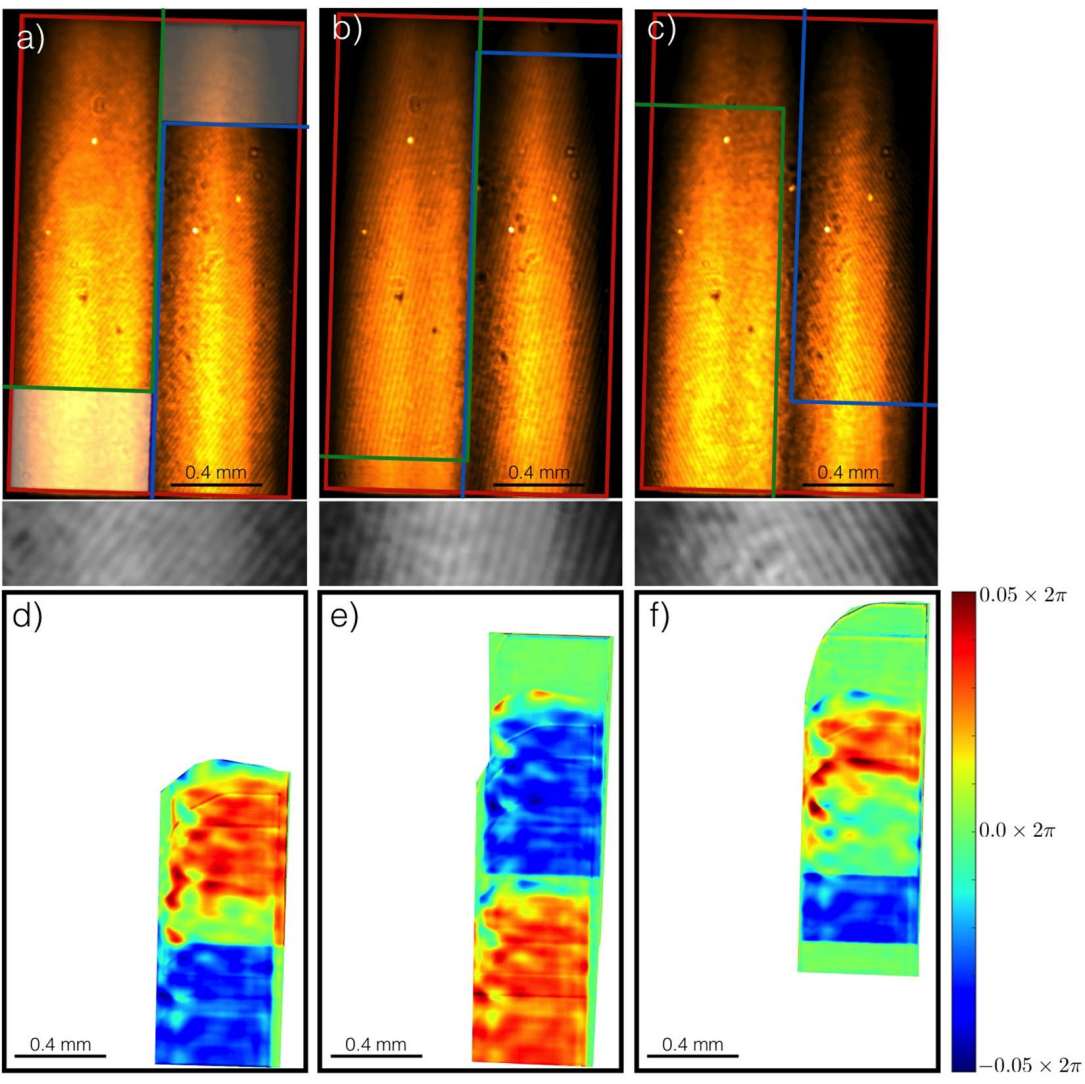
Top (a–c): the three ronchigrams used to calculate the phase of the X-ray beam, with a magnified close-up of the fringes below. The images are taken with gratings with the different spatial periods (225 nm for (a,c), and 275 nm for (b)), at the same position with respect to the focus, but with an angle of the grating with respect to the vertical of −37.9° for (a), −15.4° for (b) and 29.6° for (c). The red rectangle is the analysis mask of the zeroth order, the green and blue rectangles are the position of the −1 and +1 orders respectively. There is no interference and hence no phase information in the white shade area in (a). Bottom (d–f): False-color image of the difference (i.e. errors) between the phase derived from the Ronchigrams, and the phase derived after re-shearing the recovered wavefront of the beam. RMS error of the images is *λ*/55 for (a) and *λ*/40 for (b,c).
